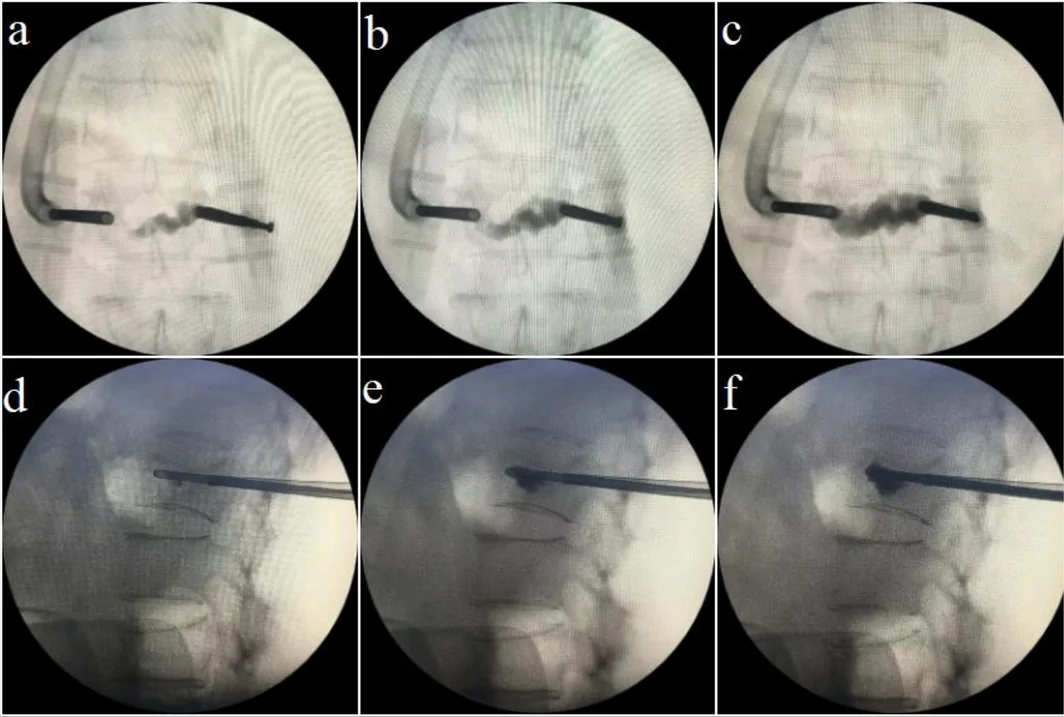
Surgical procedure of guided PVP. (a–c) intraoperative fluoroscopy (crown position). (d–f) intraoperative fluoroscopy (sagittal position). The radiograph (X‐ray) was taken on October 23, 2023.
